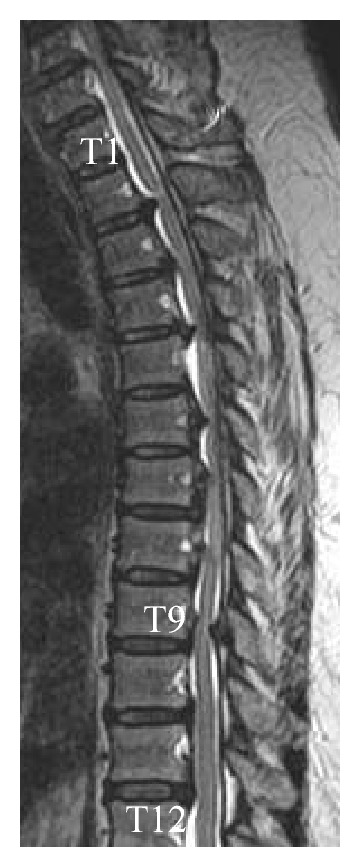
T1-weighted magnetic resonance imaging showed areas of OPLL extending from T2 to T8 and T9/T10 OYL.
